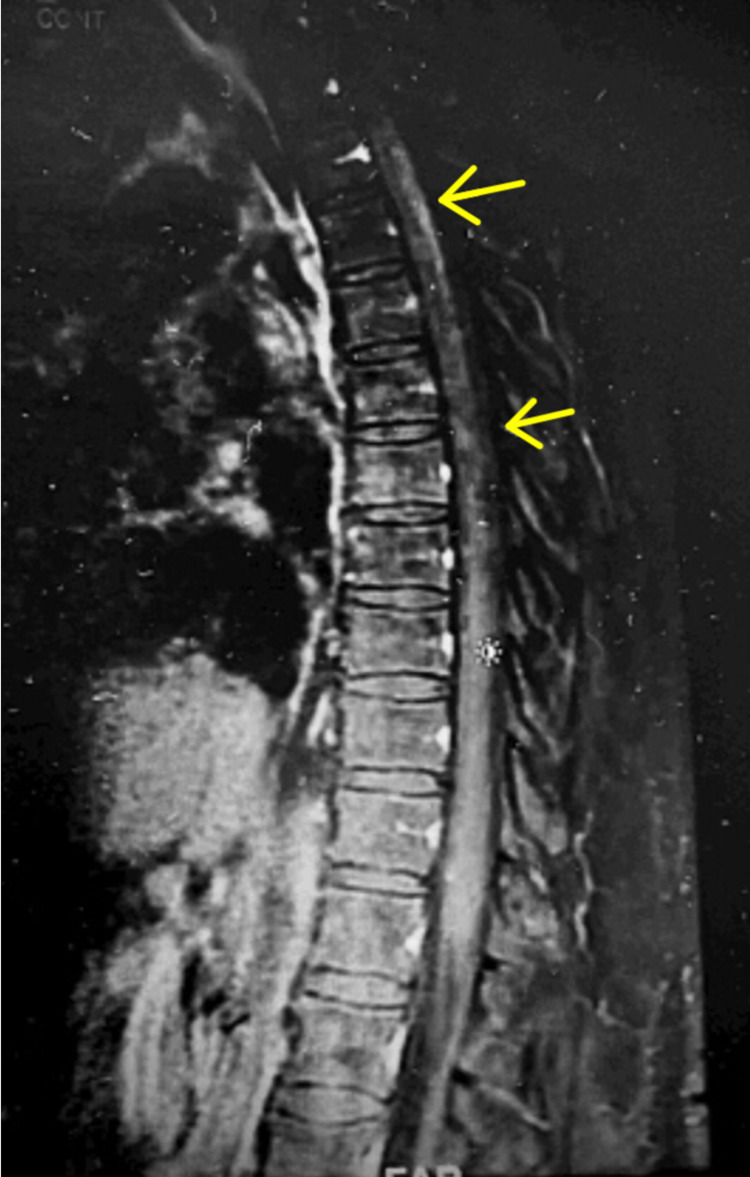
T1-weighted thoracic spine MRI post-contrast (arrows denoting “candle-guttering” appearance, characteristic of syphilitic myelitis).
